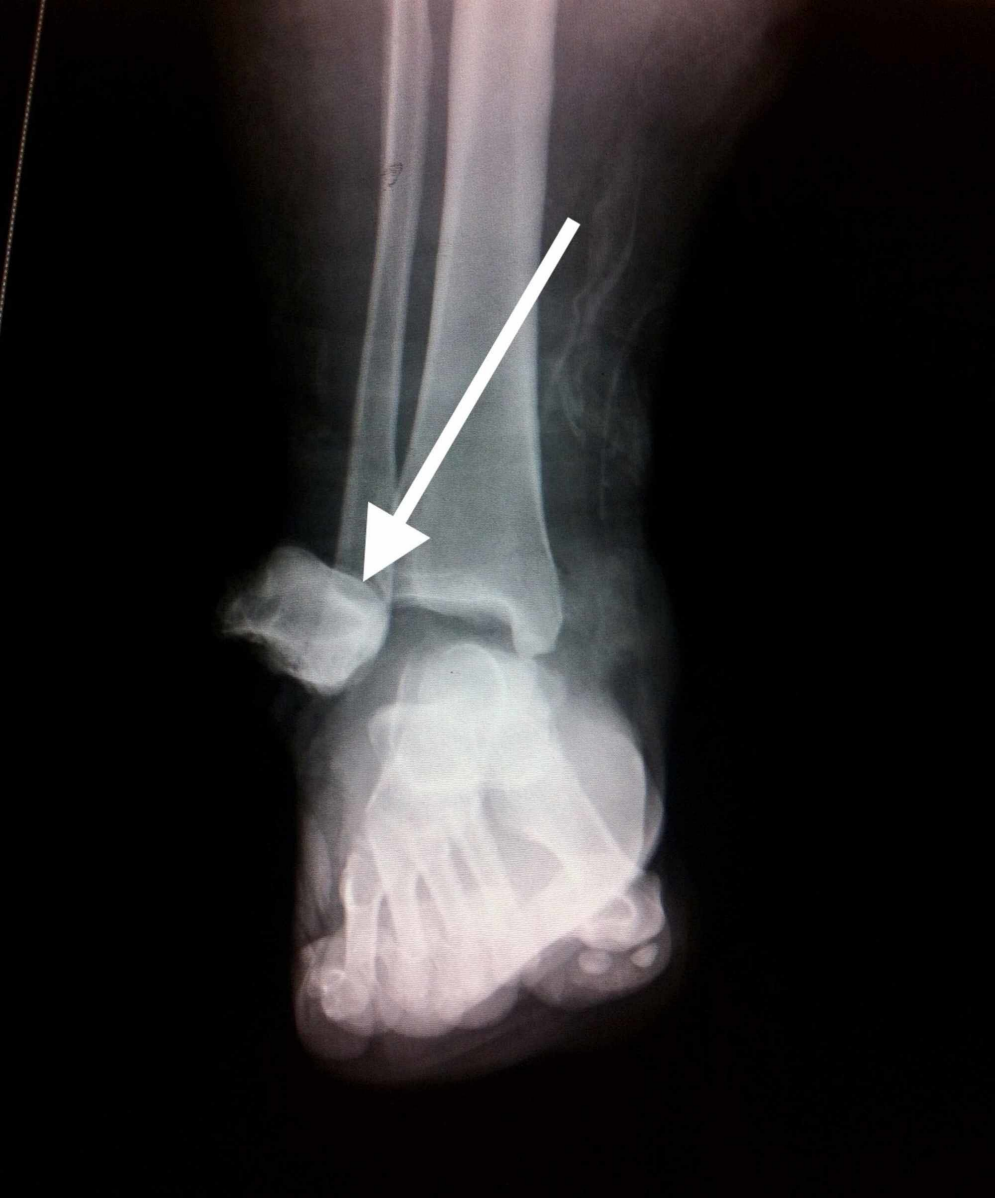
Preoperative antero-posterior radiograph of the right ankle demonstrating pan-talar dislocation (arrow)
